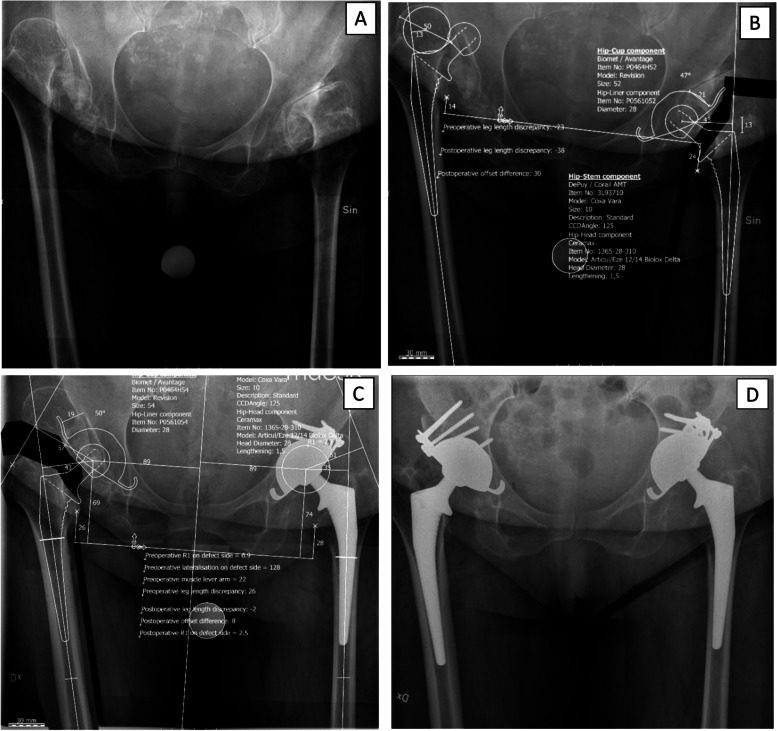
A Preoperative AP radiograph of patient with bilateral DDH: left hip Crowe type III and Hartofilakidis type B, right hip Crowe type IV and Hartofilakidis type C. B Preoperative templating of the left hip. C Preoperative templating of the right hip. D Postoperative AP radiograph after bilateral THA
